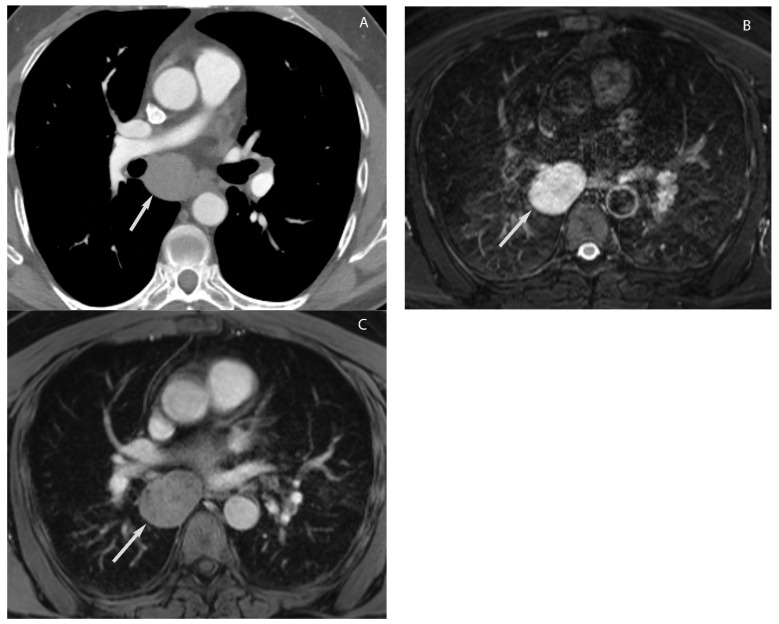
Foregut duplication cyst. CT (A) shows a well-marginated structure in the visceral mediastinum with attenuation values slightly higher than simple fluid. MRI (B,C) show high signal intensity upon T2W imaging (arrow in (B)) and no enhancement upon post-gadolinium imaging (arrow in (C)) consistent with fluid.
